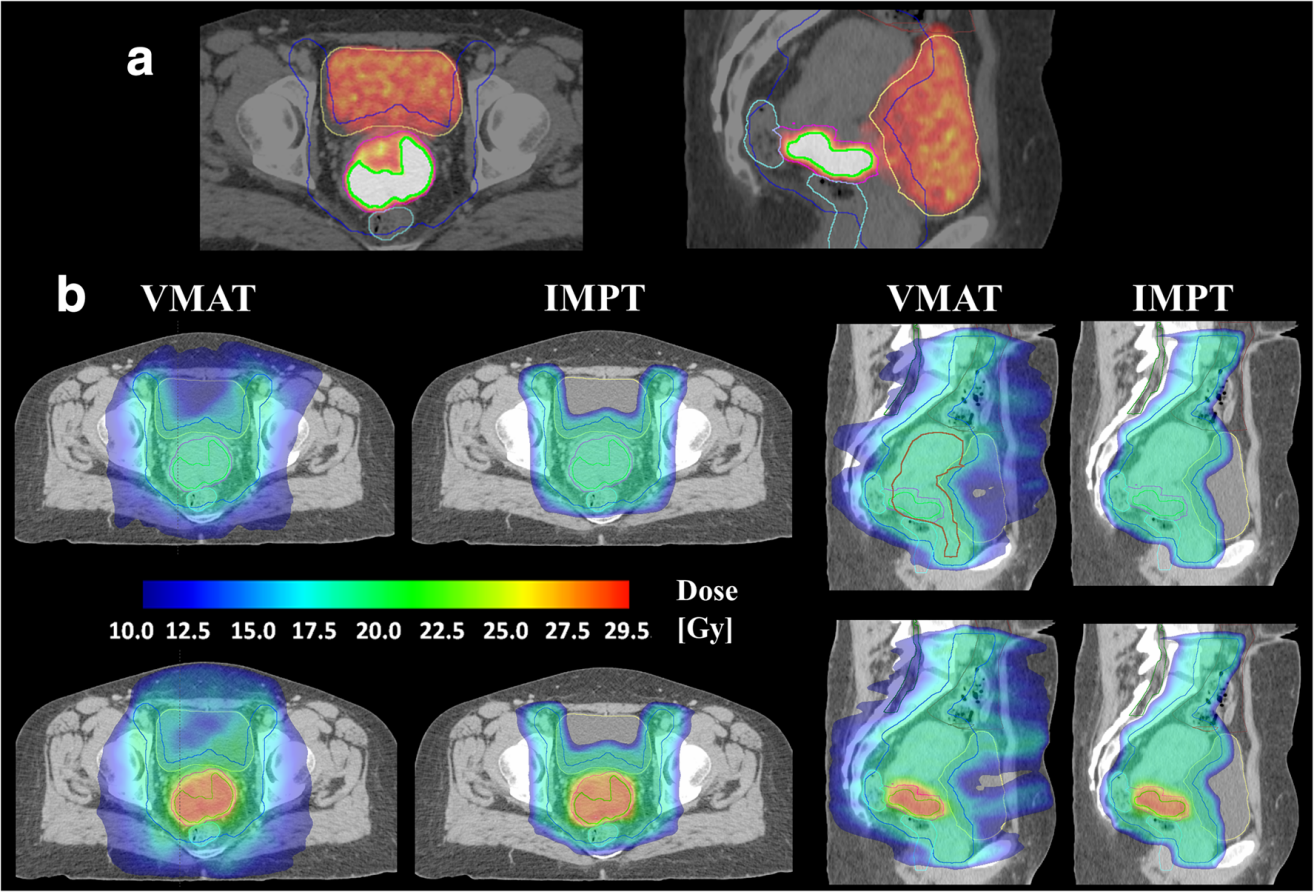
a Axial and sagittal views of the FDG PET/CT scan for one patient (GTV_tumour_ volume 66 cm^3^, MTV_50_ volume 31 cm^3^ and SUV_max_ 31). Target volumes PTV_union_ (blue), GTV_tumour_ (*pink*) and MTV_50_ (*green*) and OARs bladder (*yellow*), rectum (*light blue*) and bowel (*brow*n) outlined. b Axial and sagittal views of the obtianed dose distributions. Fractions 1–10 are shown for both VMAT and IMPT plans, comparing a standard homogenious plan (*top*) to the corresponding short-course SIB with additional 1.0 Gy per fraction to MTV_50_ (*bottom*)
